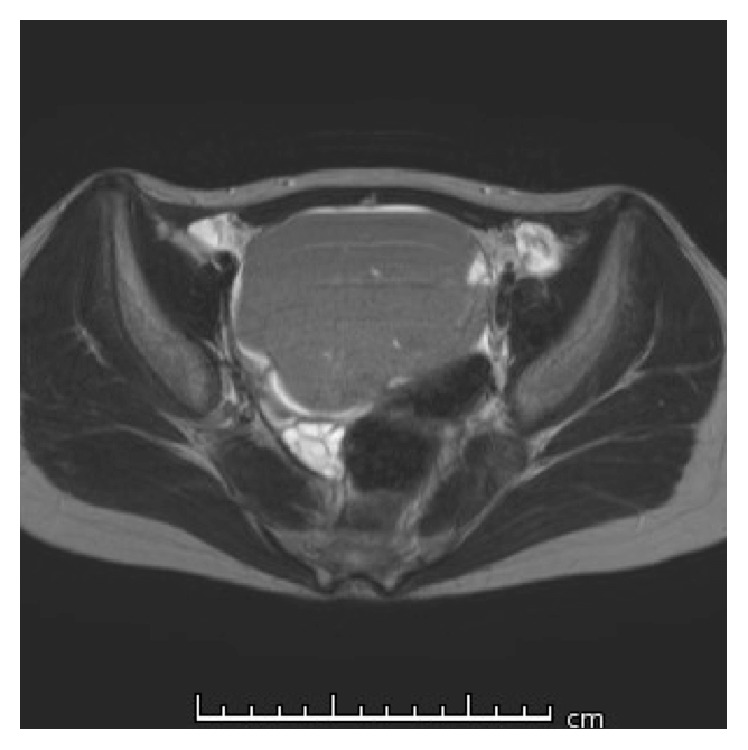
Pelvic magnetic resonance imaging (T2-weighted image) before the first surgery of Case  1. An intrapelvic tumor (9.2 × 7.6 × 7.5 cm in diameter) with high signal intensity is detected. The right ovary is normal.
